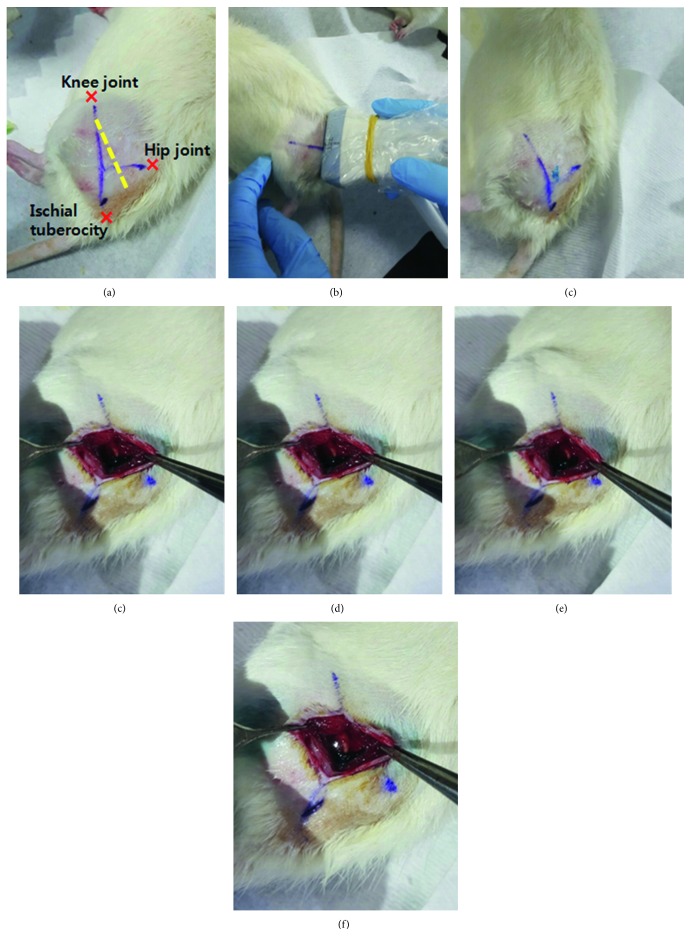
Perineural dexmedetomidine injection under ultrasound guidance. (a) Expected path of sciatic nerve marked with yellow dotted line. The sciatic nerve runs between the hip joint and the ischial tuberosity toward the knee joint. (b) Sciatic nerve detection by placement of probe obliquely or vertically on expected course. An image of the nerve as detected via ultrasound is shown in Figure 1. (c) Blue dye injection using same volume of DXM (0.08 ml) in experiment. The entry point is indicated by the blue spot. (d–f) A series of photographs of post-blue-dye-injection. After incising the skin between hip joint and ischial tuberosity and dissecting fascia and muscle, blue dye is visible around the sciatic nerve.
